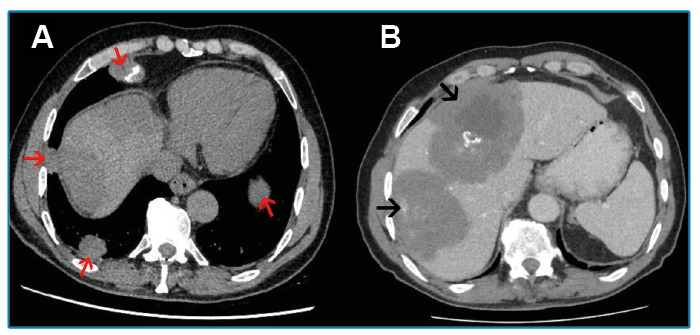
A. Thorax CT showed non-cavitary nodular lesions (red arrows) in both lungs, some of which had calcifications. B. Contrast-enhanced abdominal CT showed heterogeneous lesions with irregular contours (arrows) containing calcifications and cystic components.
